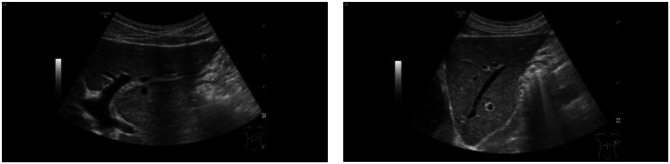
Abdominal ultrasound showed no signs of fibrosis or cirrhosis of the liver with smooth organ contour, acute-angled lower rim, homogeneous echonormal internal reflex pattern, inconspicuous vascular architecture and no focal lesions
